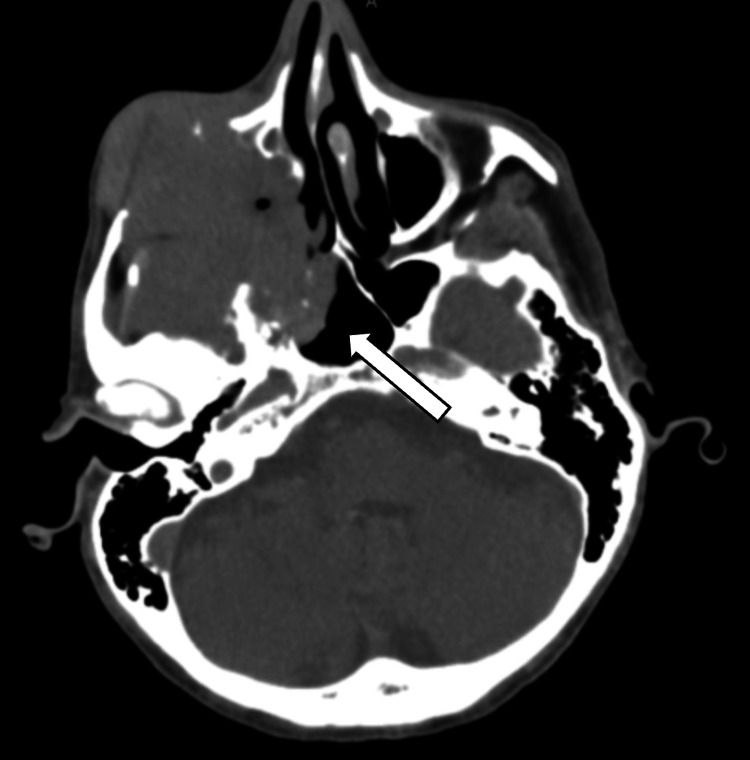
CT Brain revealing a large infiltrative mass on the right maxillary sinus. Mass centered in the right maxillary/maxillary sinus with mass effect, osseous erosion, and vascular encasement as described above. The mass invades the right anterior middle cranial fossa abutting the right inferior temporal lobe, right sphenoid sinus, and right orbit. The mass demonstrates erosion of the right maxilla, maxillary sinus walls, and multiple other osseous structures.
